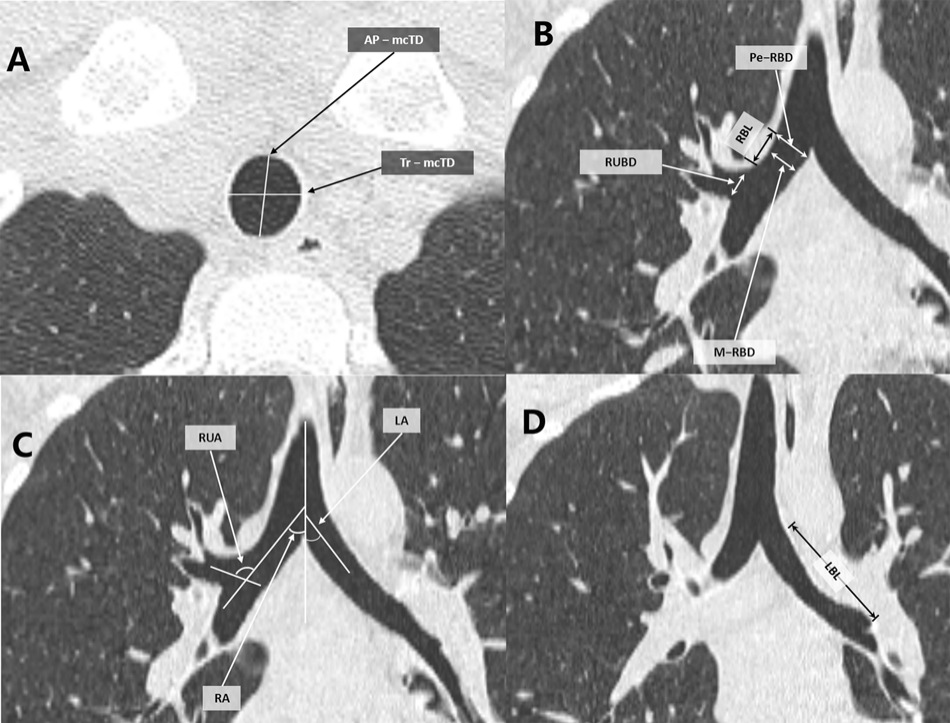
Measurements of the length, diameters and angles using CT scan and MPR. (A) AP-mcTD (Anteroposterior-Midclavicular tracheal diameter), Tr-mcTD (Transverse-Midclavicular tracheal diameter), (B) Pe-RBD (Proximal end-Right main bronchus internal diameter), RBL (Right main bronchus length), RUBD (Right upper bronchus internal diameter), M-RBD (Intermedius-Right main bronchus internal diameter), (C) LA (Left bronchial angle), RUA (Right upper bronchial angle), RA (Right bronchial angle), (D) LBL (Left main bronchus length).
